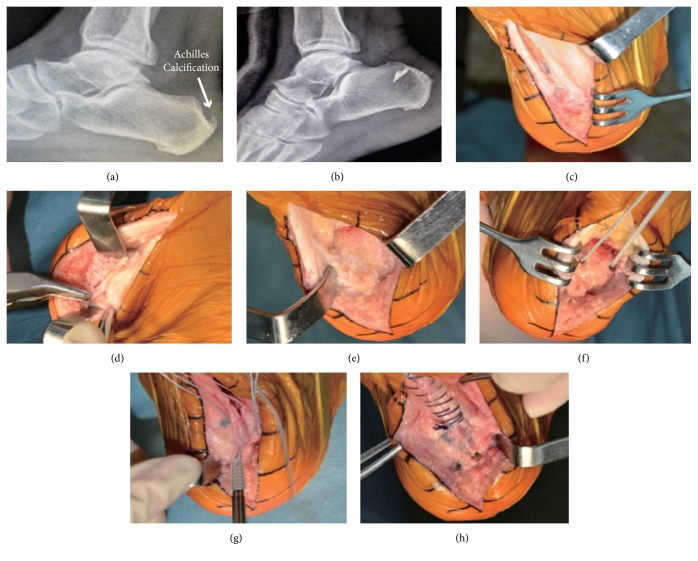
Case 1, a 67-year-old male complained of right posterior heel pain for 1 year. He could not perform the heel-rise test preoperatively and also showed uric acid level of 462 mmol/L in blood*. (a) Preoperative radiograph showed calcification in the Achilles tendon insertion. (b) Postoperative radiograph demonstrated that calcaneal calcification had been removed. (c) Central tendon-splitting approach through longitudinal incision was used to partially elevate the tendon. (d) The tendinosis portion was thoroughly debrided, and the exostosis was removed by rongeur forceps and oscillating saw. (e) After the exostosis were completely resected, the posterior calcaneal wall was levelled off. (f) The inner anchors were inserted in the proximal calcaneal tuberosity. (g) The outer anchors were inserted in the distal calcaneal tuberosity and formed the double row suture bridge. (h) Continuous suture was used to repair the split tendon.
